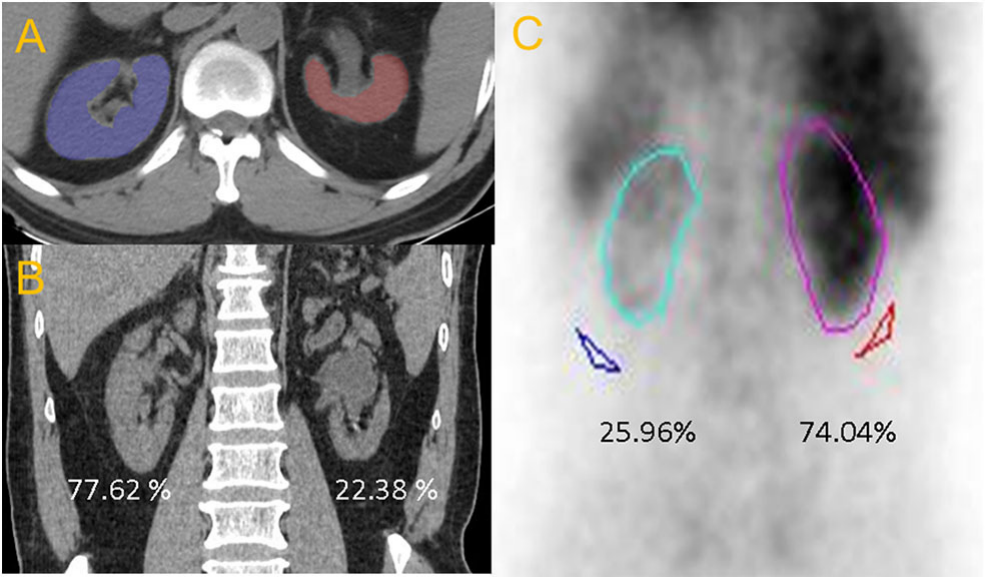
(A) Axial non-enhanced CT image. (B) The percentage of CT renal volume for right and left kidney were 77.62 and 22.38%, respectively. (C) The differential renal function from RG of right and left kidney were 74.04 and 25.96%, respectively.
